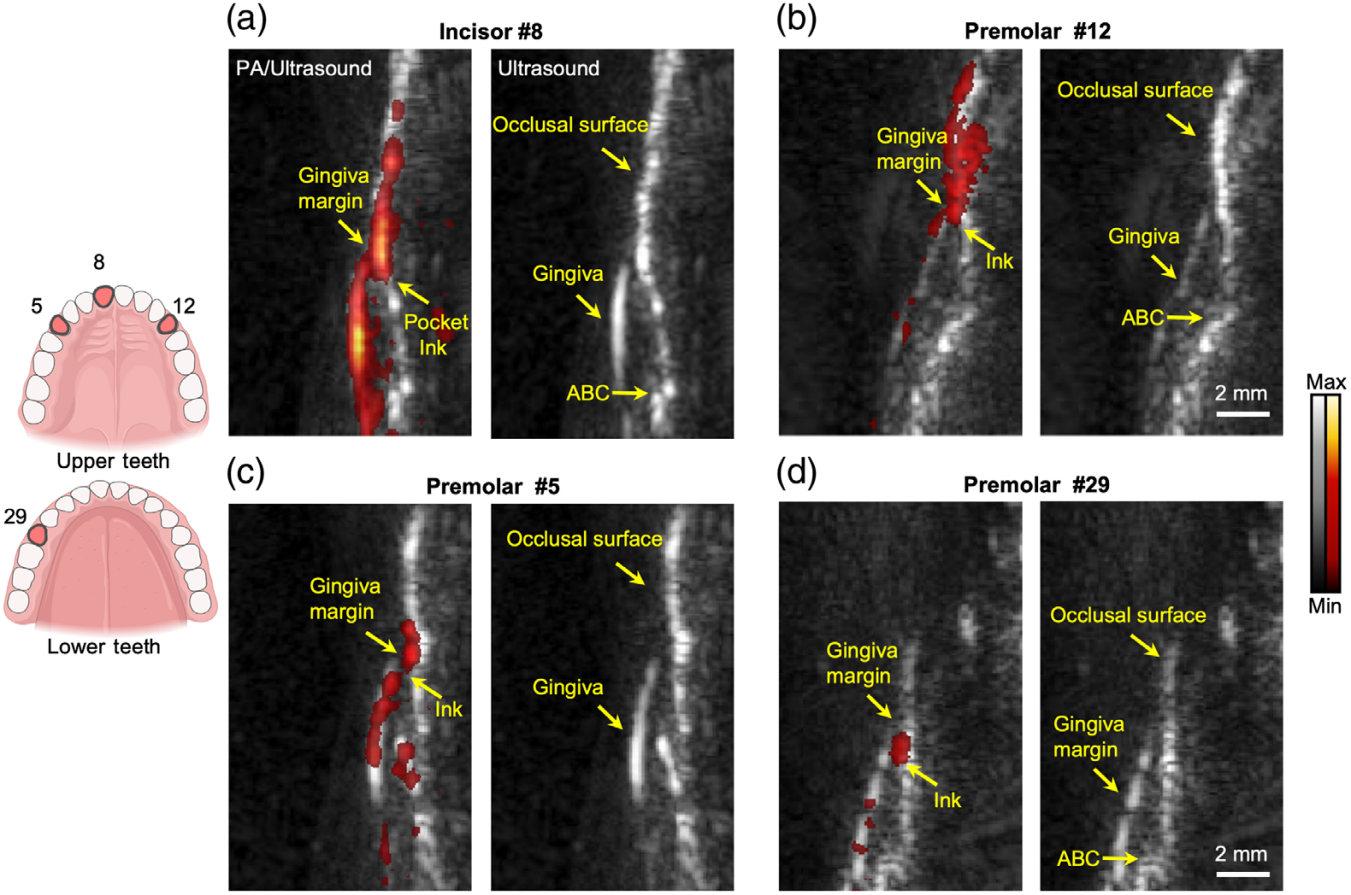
Photoacoustic/ultrasound images of four human teeth: (a) incisor #8, (b) pre-molar #12, (c) pre-molar #5, and (d) pre-molar #29. Cuttlefish ink contrast agent labels the periodontal pockets (left panels). Photoacoustic images in red are overlaid to the ultrasound images in gray. Right panels are the ultrasound images, correspondingly. Ultrasound images show the common structure of the tooth. Photoacoustic images show the position of the contrast agent.
